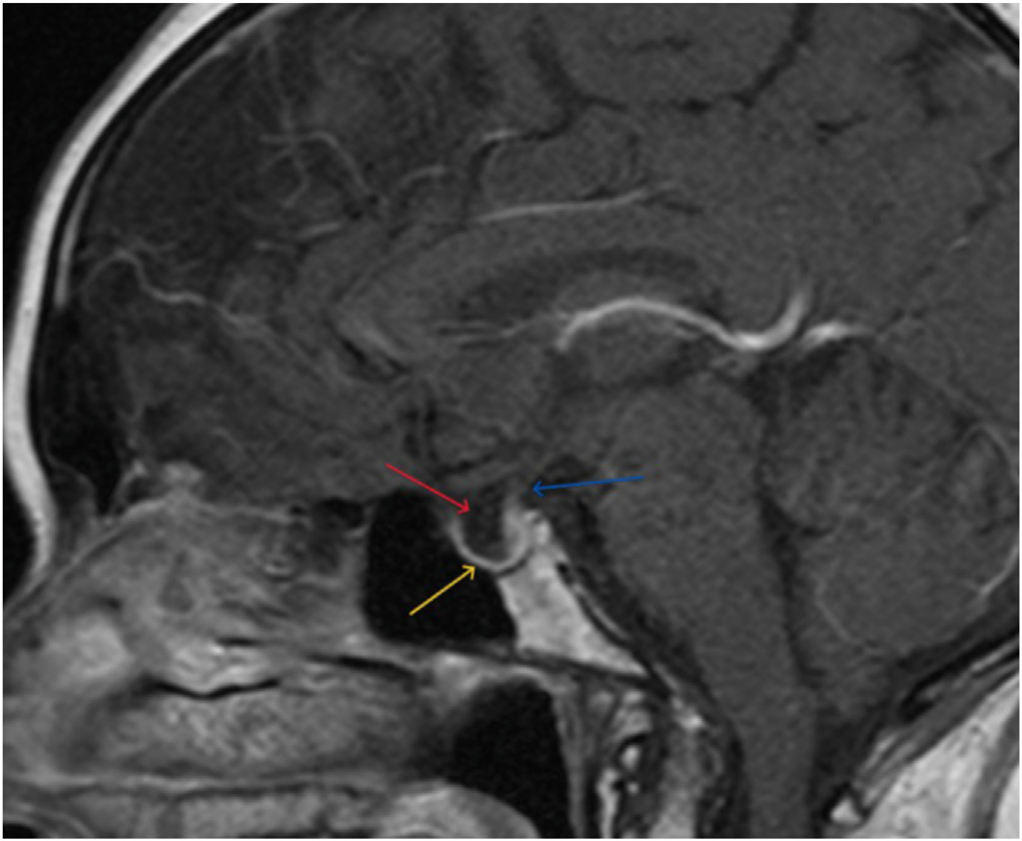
Sagittal T1-weighted postcontrast MRI of the pituitary gland demonstrating an empty sella with sellar ballooning. The sella turcica is enlarged and filled with cerebrospinal fluid (red arrow), causing marked flattening and thinning (yellow arrow) of the pituitary gland (measuring approximately 1 mm) along the sellar floor. The infundibulum (blue arrow) is noted to be stretched superiorly and deviated posteriorly. *MRI* = magnetic resonance imaging.
